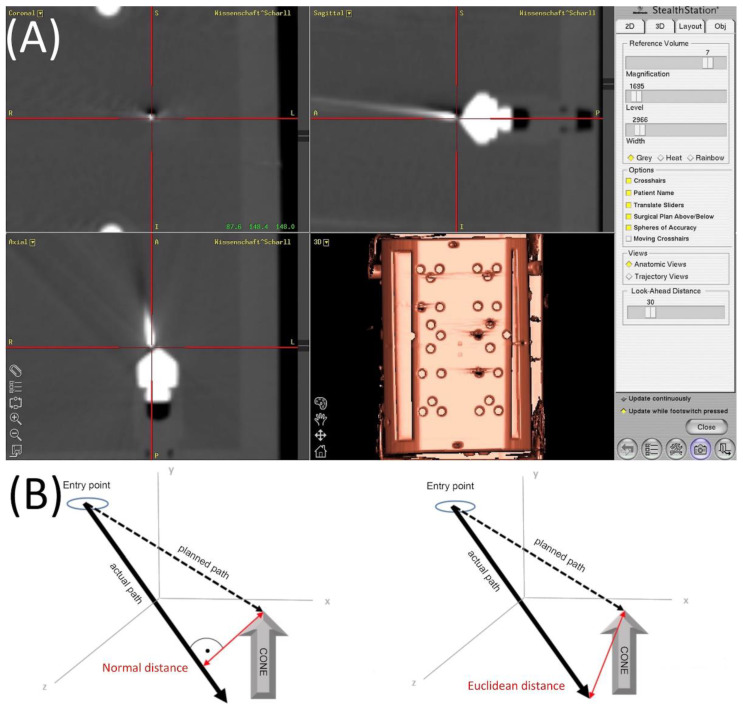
(A) Screenshot of the Medtronic Stealth Station Treon to evaluate the needle positioning accuracy with the control CT-data. The x, y and z coordinates (displayed in the left upper quadrant) were measured by positioning the crosshairs in all three planes. The data was then transferred to an Excel spreadsheet for the calculation of the Euclidean and the lateral positioning error. (B) The Euclidean distance determines the gap between two points in a multidimensional space. It is calculated using the coordinates of the actual position of the needle tip and the target point, indicating the deviation in the direction of the needle placement. The normal distance describes the shortest possible distance between a point and a straight line.
